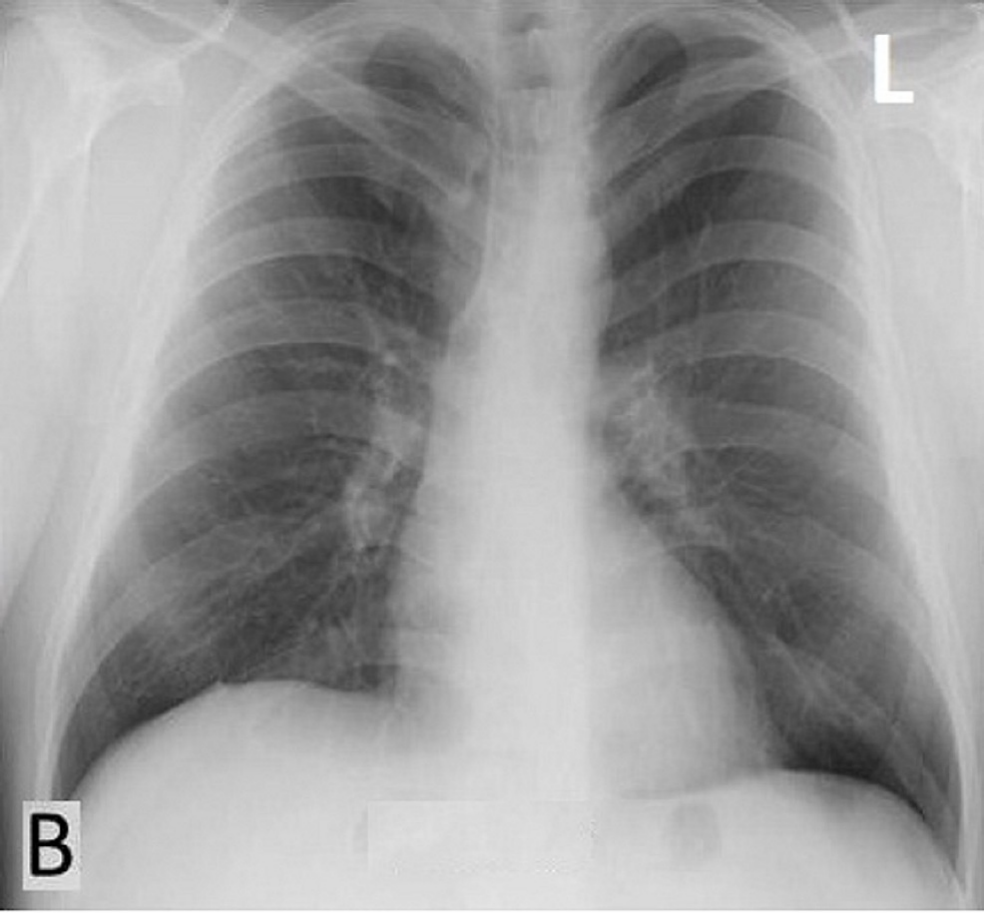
Follow-up chest X-ray (B) 30 days after onset of symptoms showed complete resolution of bilateral lung opacities.
